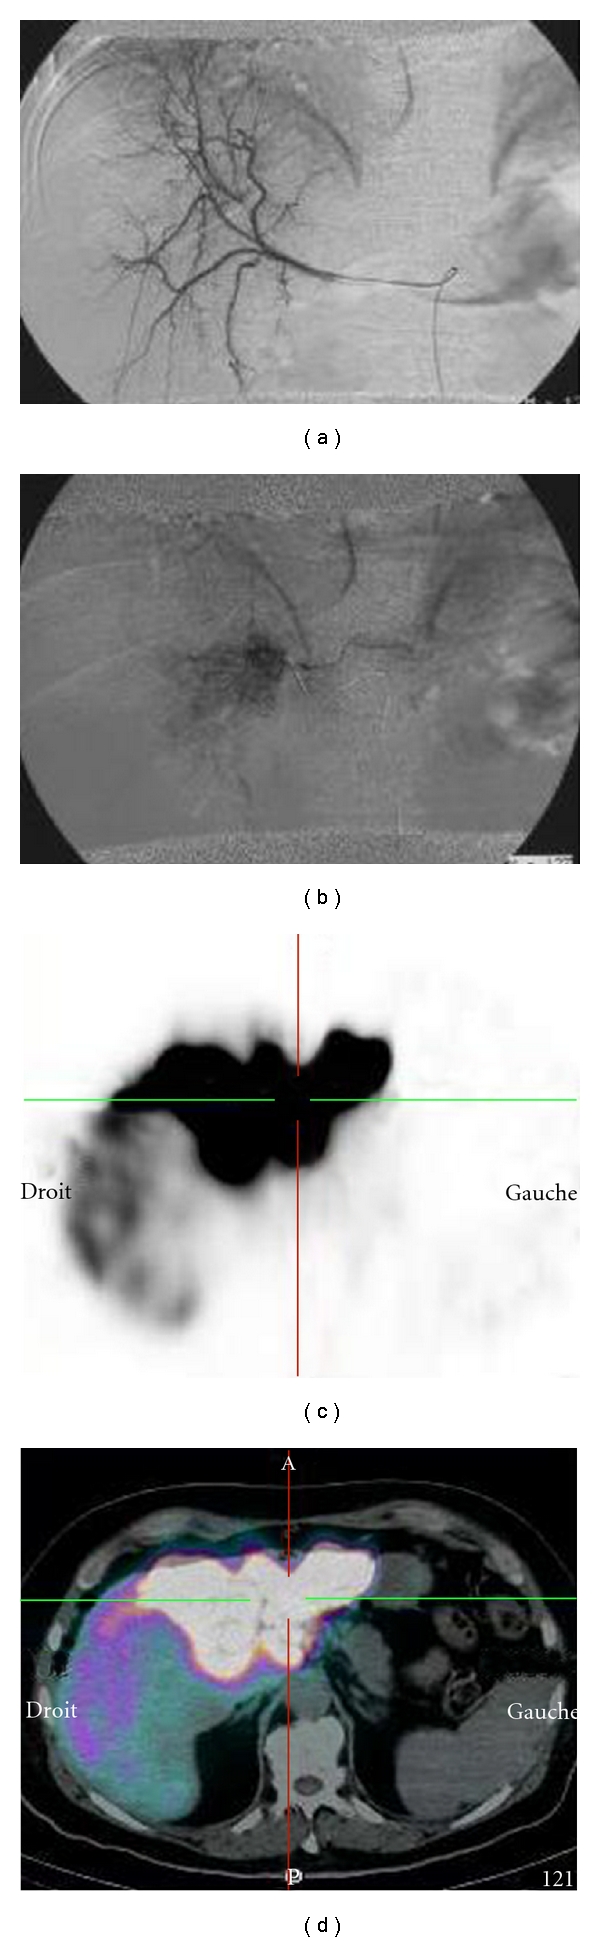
Case report: angiographic and scintigraphic data; right selective angiography (a), selective angiography of the common hepatic artery vascularizing segment IV and the left liver (b), SPECT (c) and SPECT/CT (d) after injection of the MAA at the level of the central hepatic artery revealing an unexpected uptake in the right liver, in addition to the expected uptake of segment IV and left liver (whole liver distribution, volume 1829cc).
